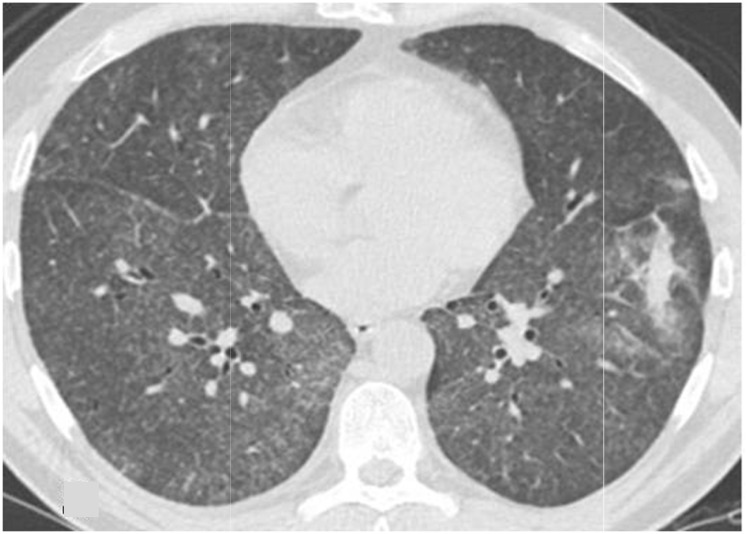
High resolution CT scan of the chest, showing diffuse and bilateral hazy increase of lung density (“ground glass” opacity), a left lower lobe consolidation, multiple fine diffuse nodules bilaterally, suggestive for miliary lung disease.
